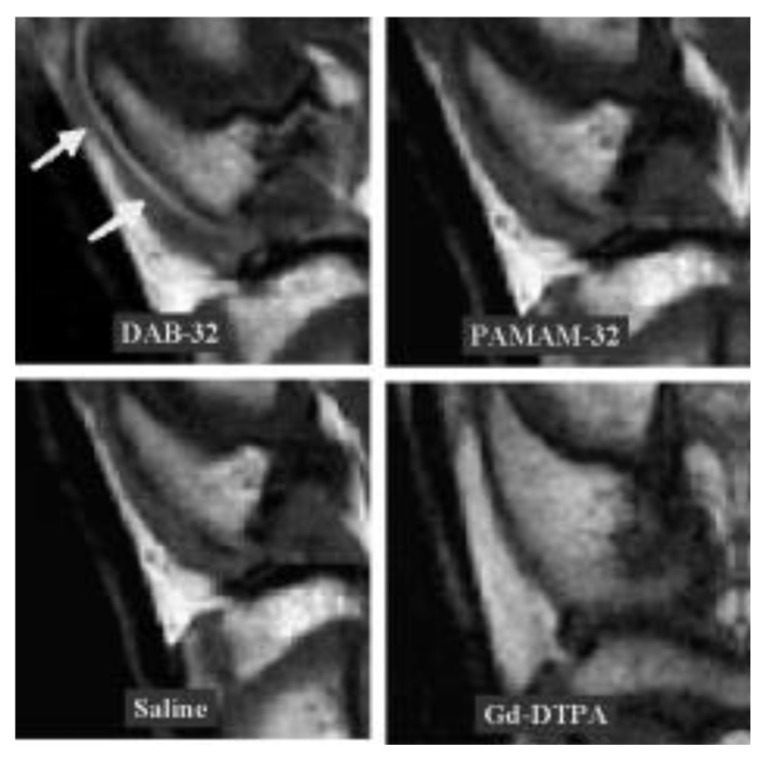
Sagittal T1-weighted images of stifle joints obtained 3 h after intra-articular injection of the agents. Arrows designate cartilage enhancement with DAB-32. Joint with PAMAM-32 unenhanced [240]. (Posted with the author’s permission).
